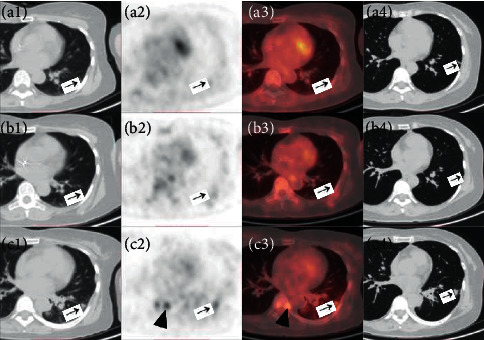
A 45-year-old female with rectal cancer underwent surgery. ^18^F-FDG PET/CT (a1-3) scan showed an increased FDG uptake in the left lower lobe of the lung (black arrow). (SUVmax = 1.9). HRCT (a4) revealed a strip-like thickening of the corresponding pulmonary vessel. The patients received systemic antineoplastic therapy and were reexamined 3 months later. PET/CT (b1-3) and HRCT (b4) scans showed that the pathological pulmonary vessel and metabolism basically returned to normal. After 11 months, ^18^F-FDG PET/CT (c1-3) and HRCT (c4) found that the pulmonary vessel was markedly enlarged with increased metabolism, and new bone metastasis appeared (black triangle) (SUVmax = 3.5).
